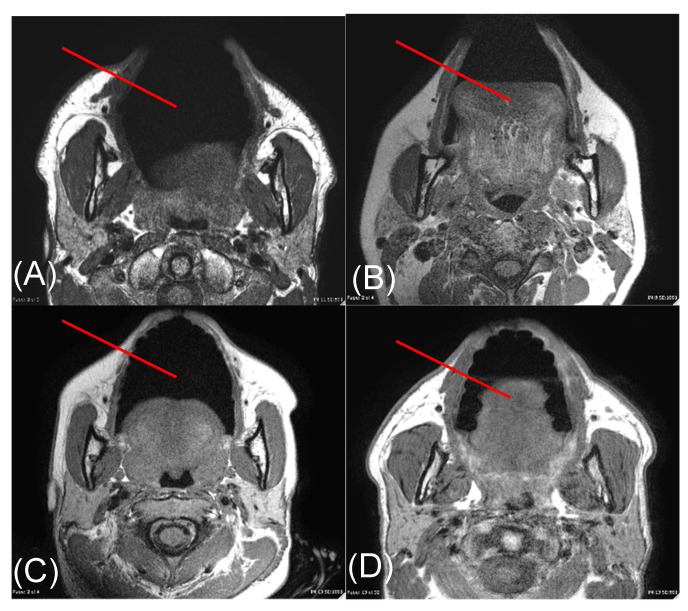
Axial T1-weighted MR images through the face acquired from subjects 1 (A), 2 (B), 3 (C), and 4 (D). The red line on each image depicts the trajectory along which a representative light beam travels during transmission measurements. Measurements were made along this red line from the surface of the skin to the surface of the buccal mucosa.
